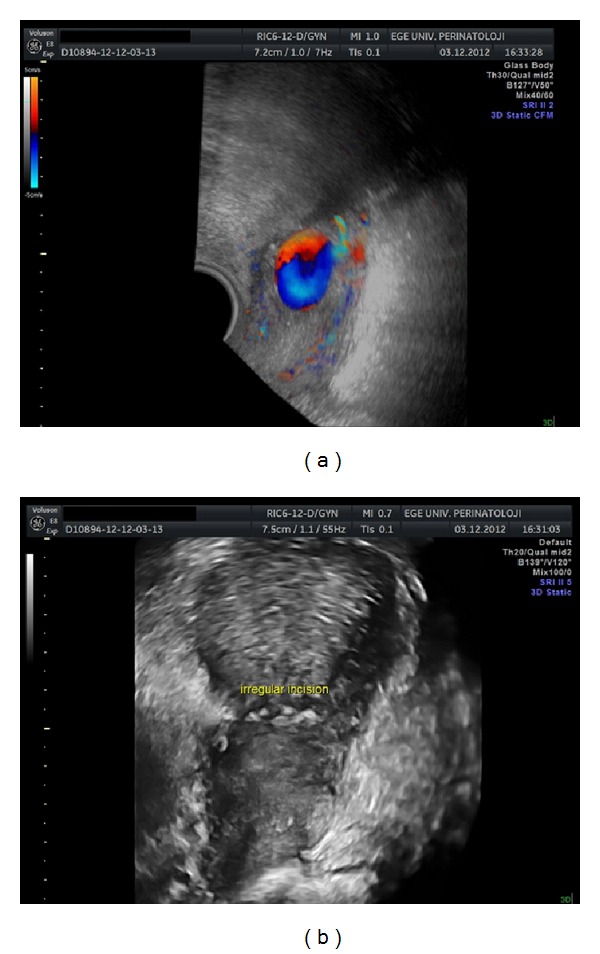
Three-dimensional (3D) sonography or 3D power Doppler mode showed the same cystic mass with a suspicion of irregular incisional track and the highly vascularized cystic mass anastomosed to the uterine vessels.
